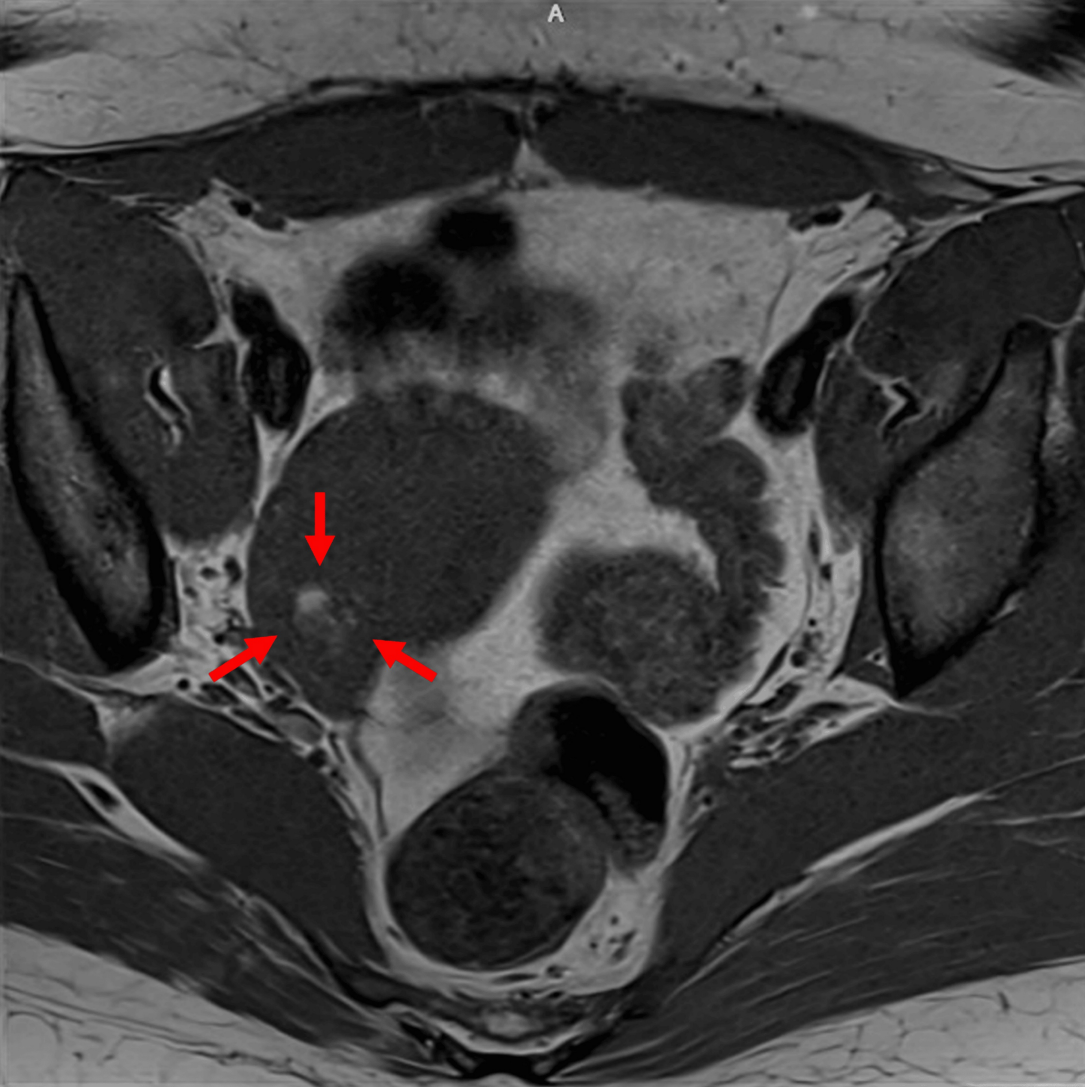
Radiological findings of ovarian teratoma. Magnetic resonance imaging showed a small fatty lesion within the central right ovary without enhancement (red arrow).
